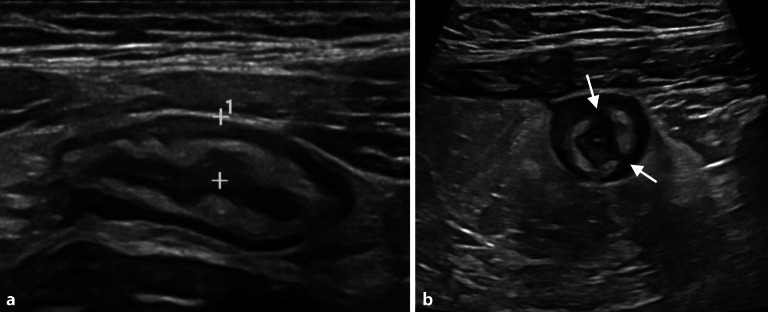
Die Sonographie zeigt eine deutliche Wandverdickung auf > 3 mm (a, *Distanzmarken*) mit einer Verdickung der echoreichen Submukosa mit fokalen hypoechogenen Unterbrechungen der Schichtung (b, *Pfeile*), hinweisend auf das Vorliegen einer aktiven Entzündung
